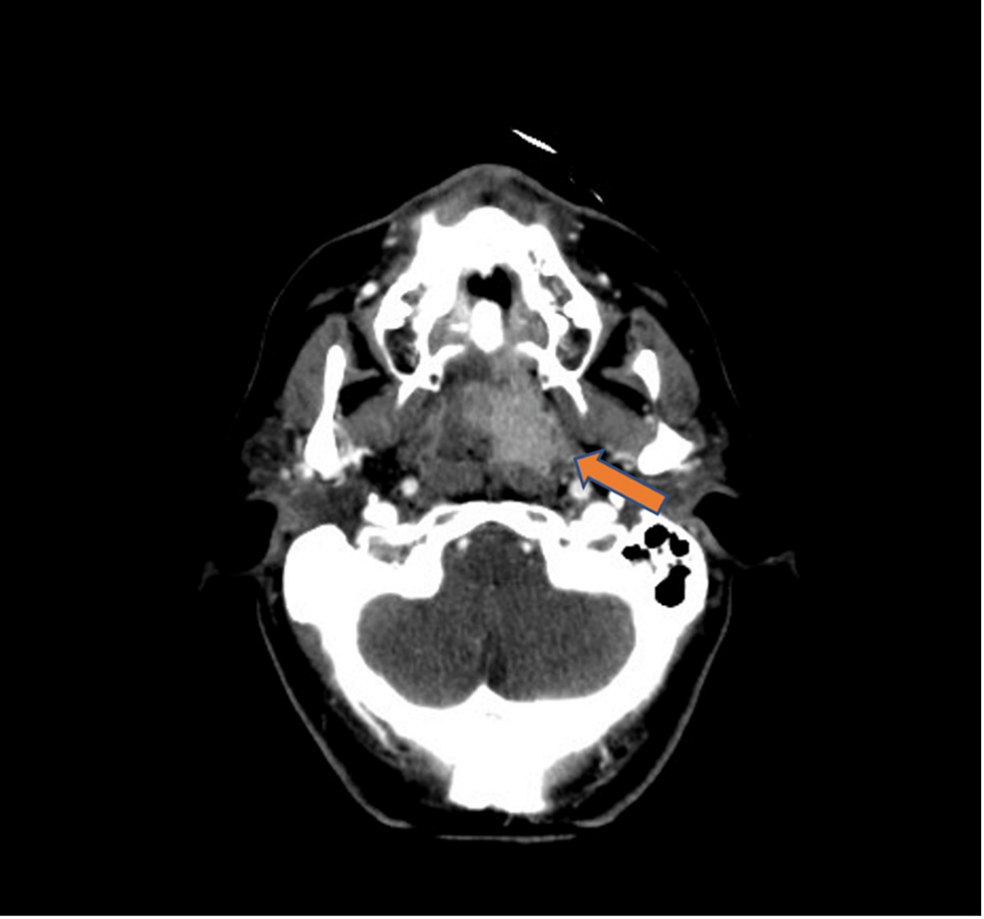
Computed tomography showing a contrast-enhancing mass in the left nasopharynx (arrow).
